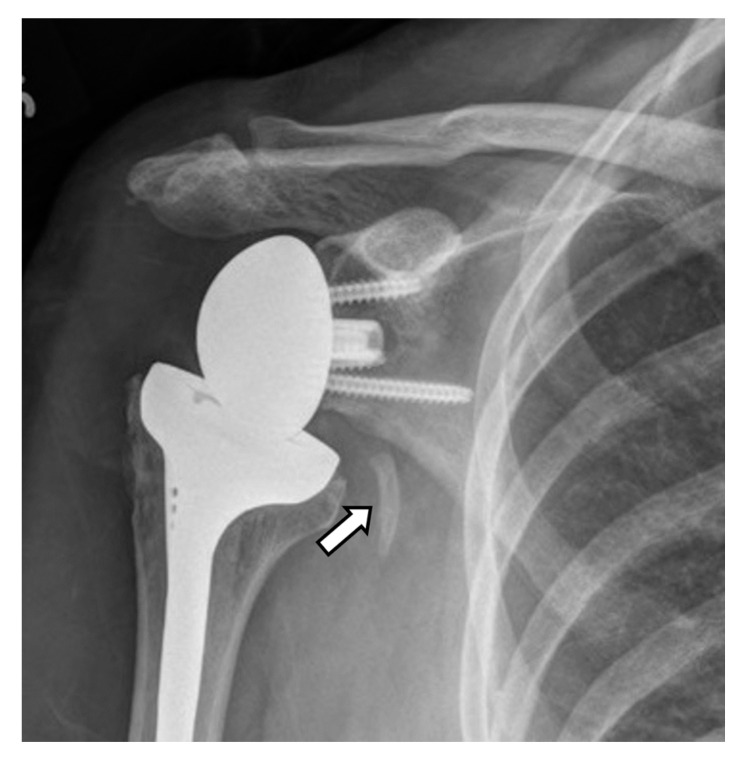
Frontal radiograph shows heterotopic ossification (arrow) along the proximal medial aspect of the humeral stem of an RTSA.
